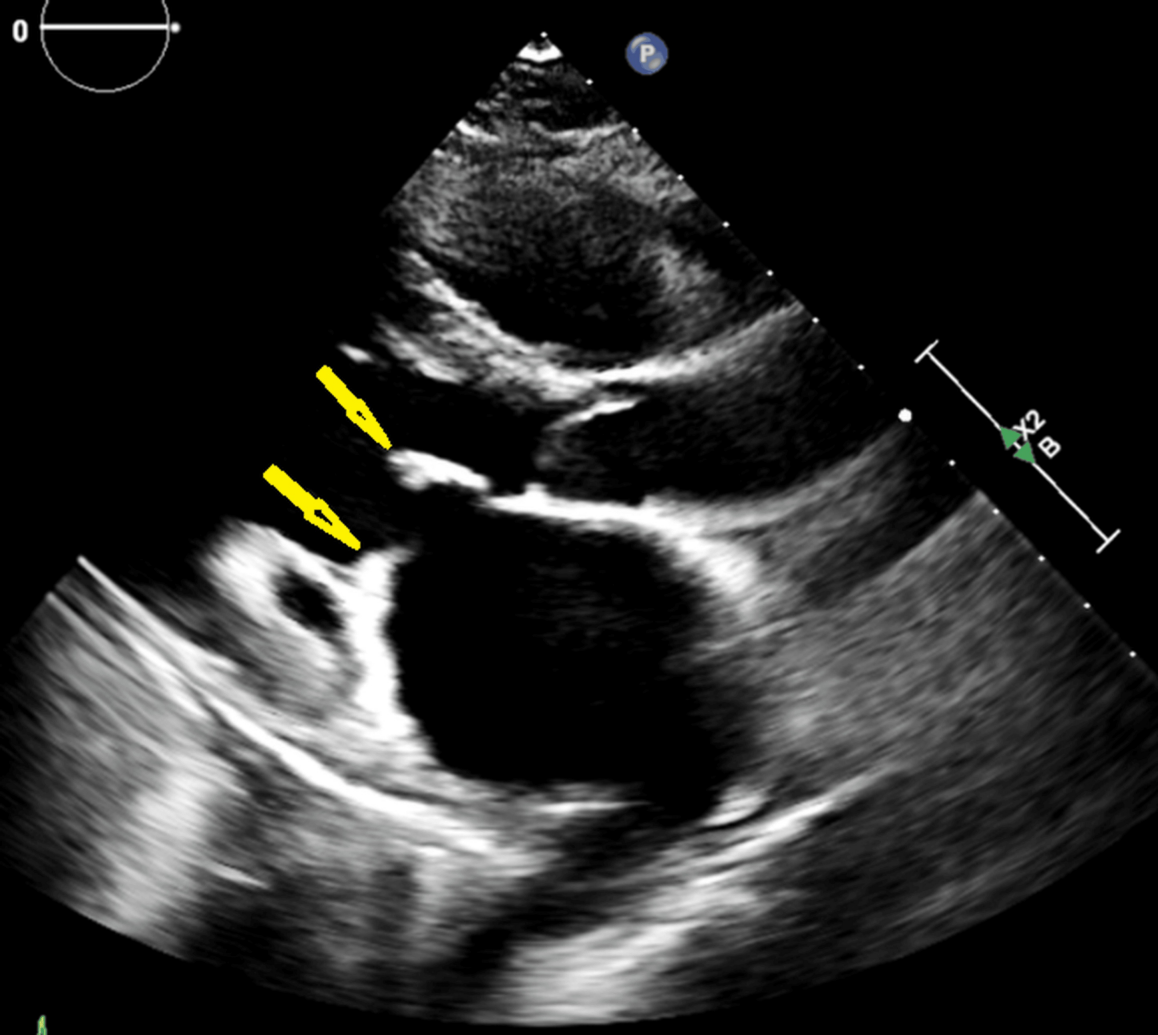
Follow-up transthoracic echocardiography Follow-up transthoracic echocardiography demonstrating persistent mitral valve vegetations (yellow arrows) with partial improvement in left ventricular systolic function following immunosuppressive therapy.
